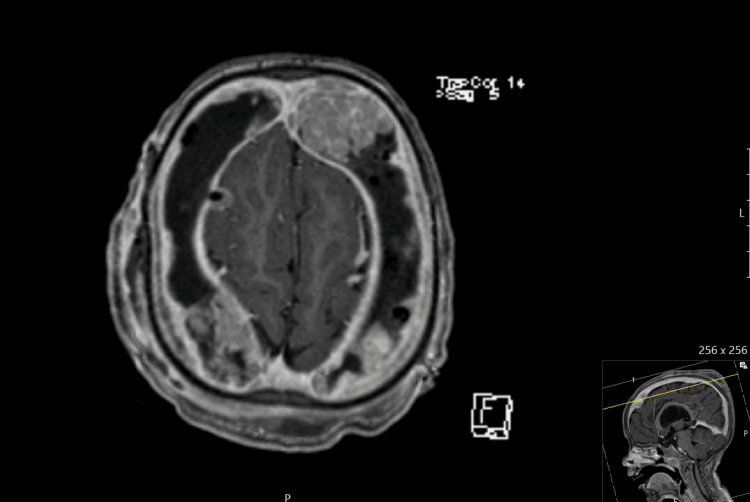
Contrast-enhanced axial T1 MRI image showing subdural space occupied with multiple nodular masses.
